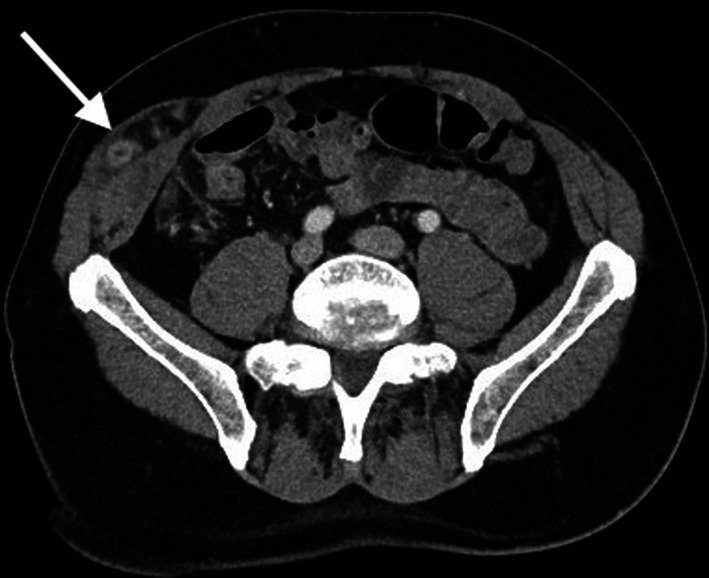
Axial computed tomography of the abdomen and pelvis showing appendicitis within a Spigelian hernia.
